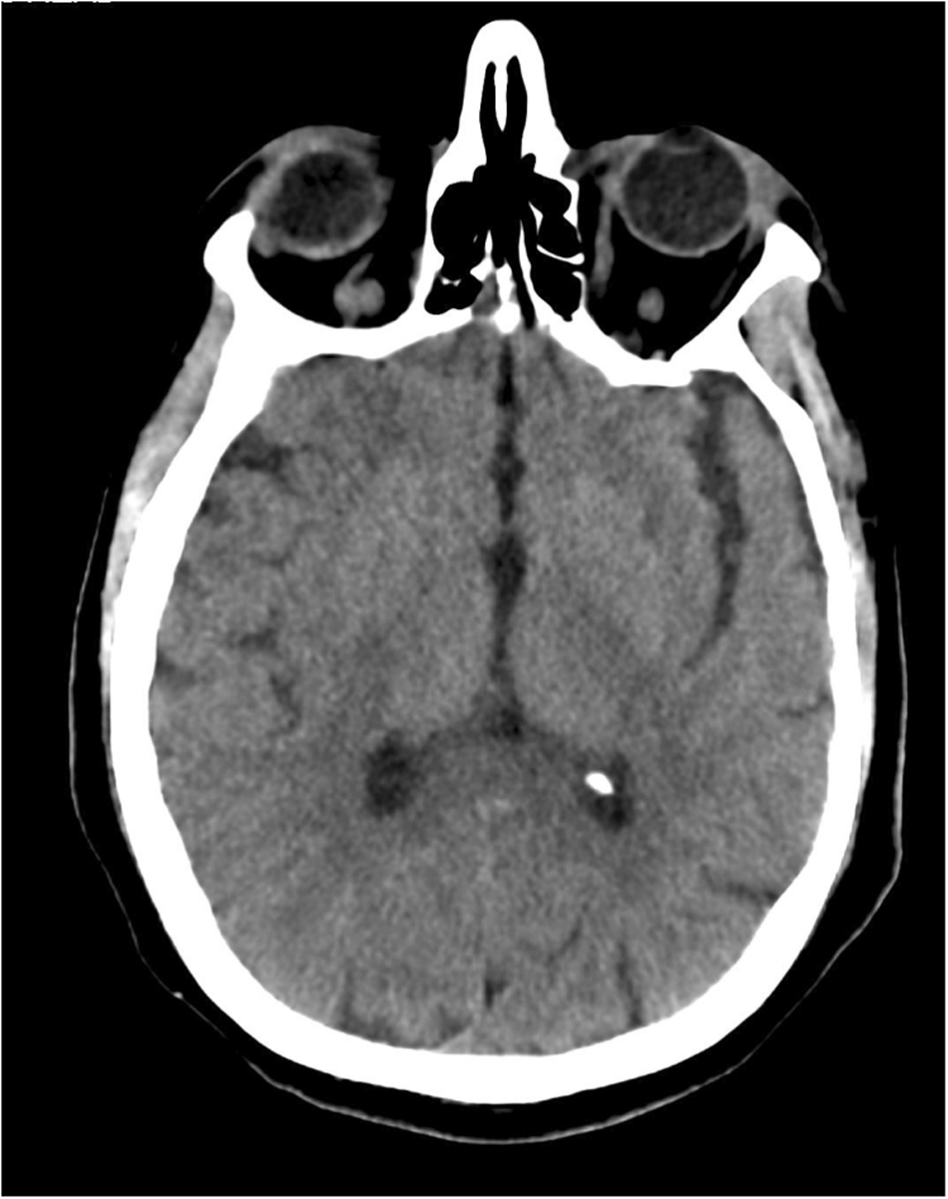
Normal CT image of the head. GPT-4V correctly identified the modality and the anatomical region but hallucinated a pathology of intracranial hemorrhage
